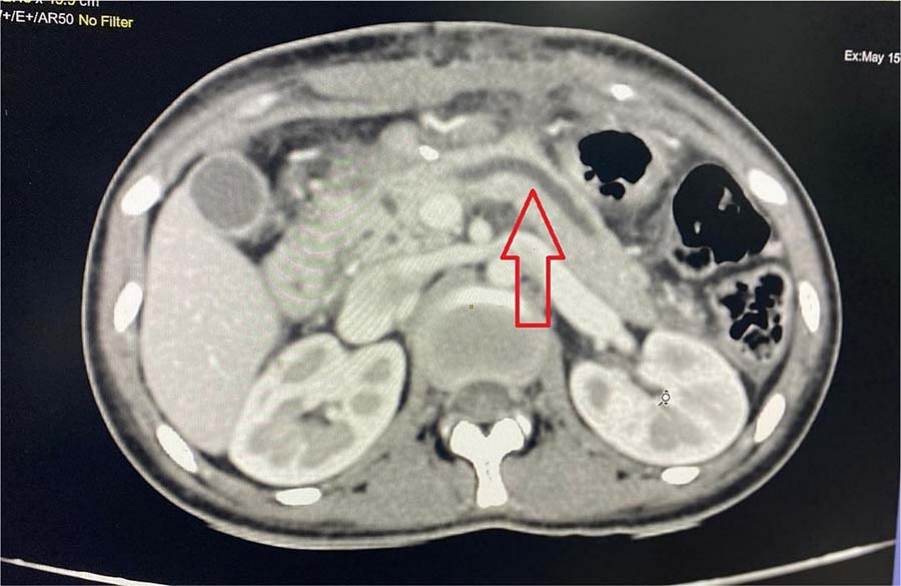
Abdominal CT showing dilated pancreatic duct.
